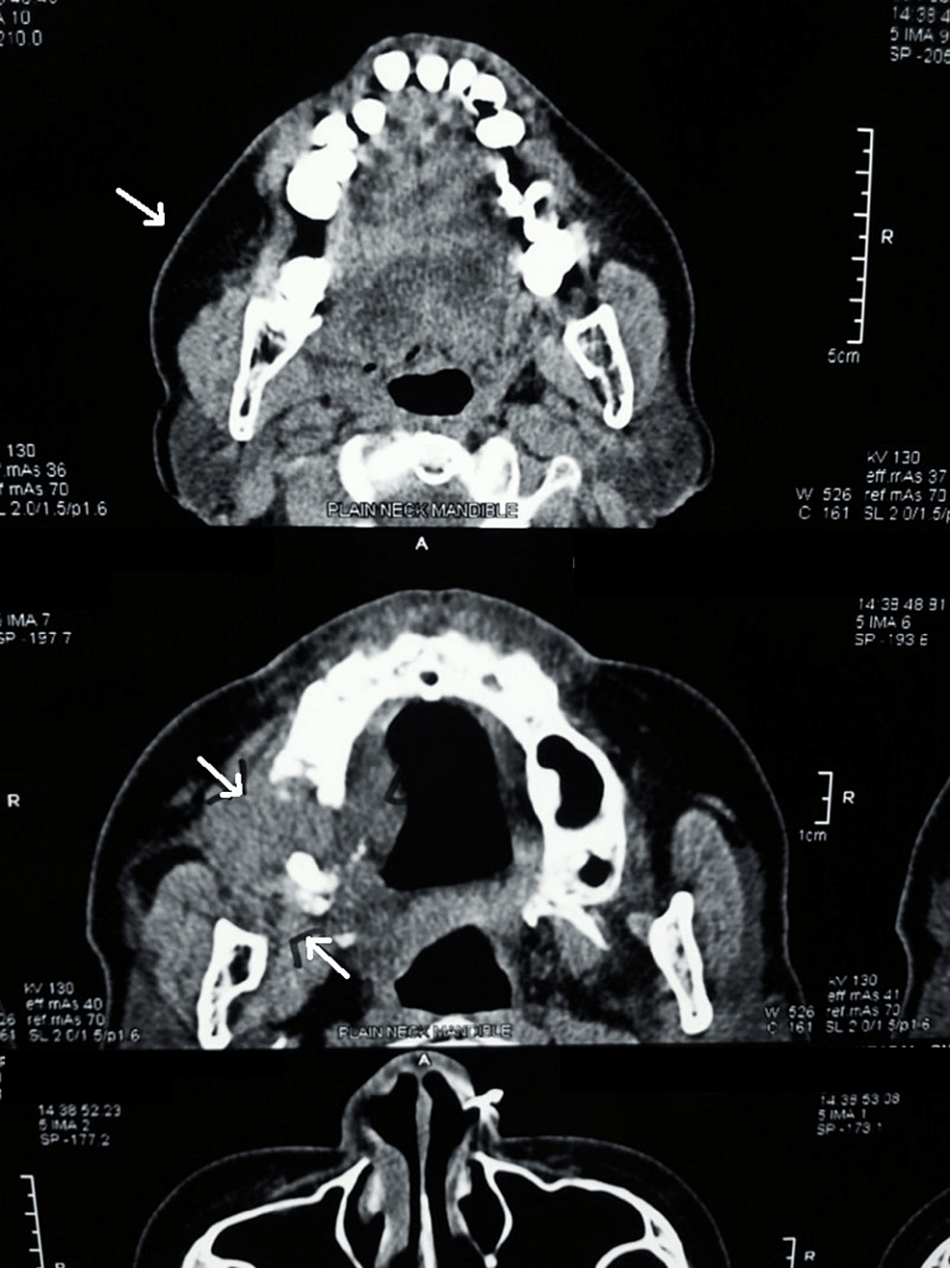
CT scan showing hypodense soft tissue density on the upper right alveolar arch.
